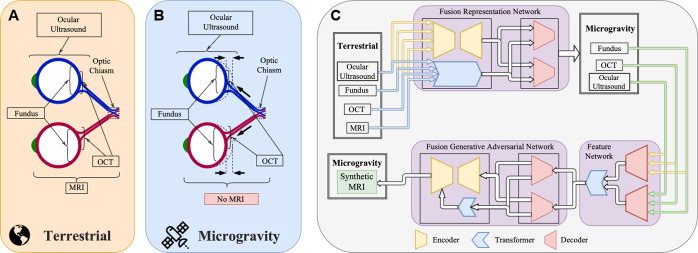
Leveraging artificial intelligence (AI) to study the pathophysiology behind SANS. A Terrestrial mechanism such as optical coherence tomography (OCT), orbital ultrasound (OU), fundus photography, and magnetic resonance imaging (MRI) perform various measurements on the structure of the human visual pathways. B Post-flight imaging has shown that shifts of the brain and the optic chiasm, among other things, produce ocular structural changes such as globe flattening, choroidal folds, and optic disc edema. Unfortunately, some imaging modalities such as MRI are not conducive to be deployed in-flight. C Sophisticated artificial intelligence (AI) techniques such as generative adversarial networks (GANs) have shown promise in their ability to fuse information from multiple data modalities to produce effective representation of the data shared across these modalities. Encoders take encodes from the data domain into the latent/feature space, decoders decode from the latent/feature space back into the data space, and transformers aids with fusion and incorporating temporal and spatial correlations in the data. Inspired by these advances, GAN architectures hold the key in establishing a fusion of representative features among various modalities pertinent to SANS in order to produce imaging data unavailable in-flight. This provides a new era in studying SANS and the risk of its progression.
